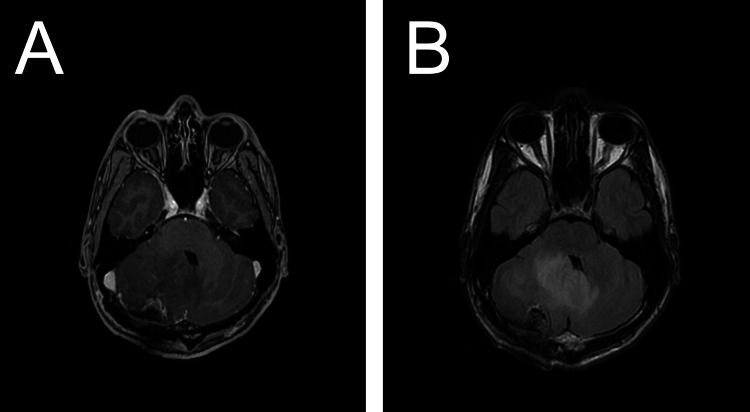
Post-operative MRI shows gross total resection of the right cerebellar mass with pathology consistent with hemangioblastoma. (A) Post-operative axial T1-weighted contrast-enhanced image demonstrates a resection cavity lined by thin, linear enhancement consistent with post-operative changes. (B) Post-operative axial T2-FLAIR weighted image demonstrates resection cavity with persistent mass effect. FLAIR: fluid-attenuated inversion recovery
